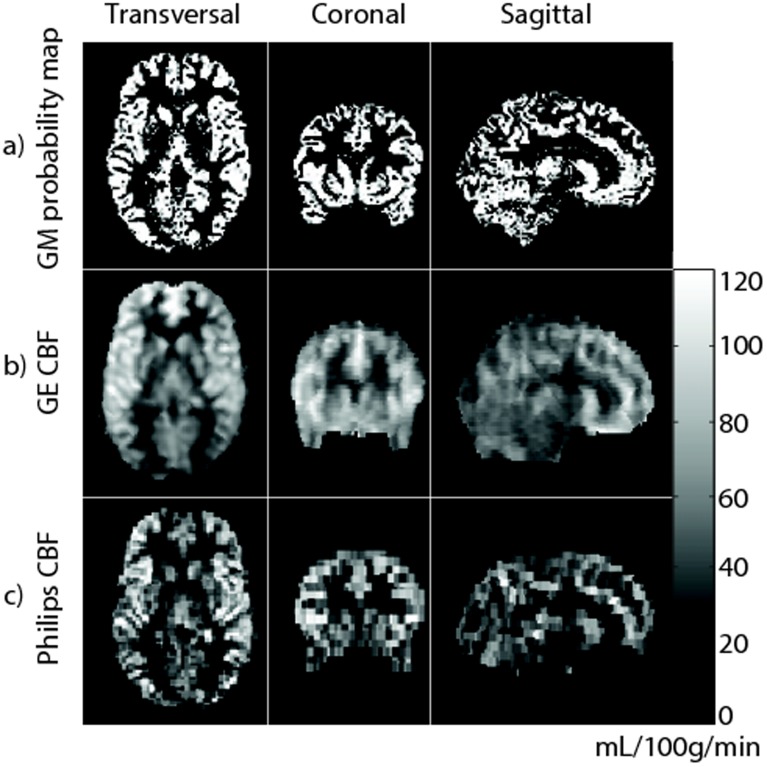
Cerebral blood flow maps of a representative subject of GE (b) and Philips (c), as compared to gray matter (GM) tissue probability map (a; for this example the GE 3D T1-weighted image was used). Maps are registered, re-sliced, skull-stripped and shown in native space.
